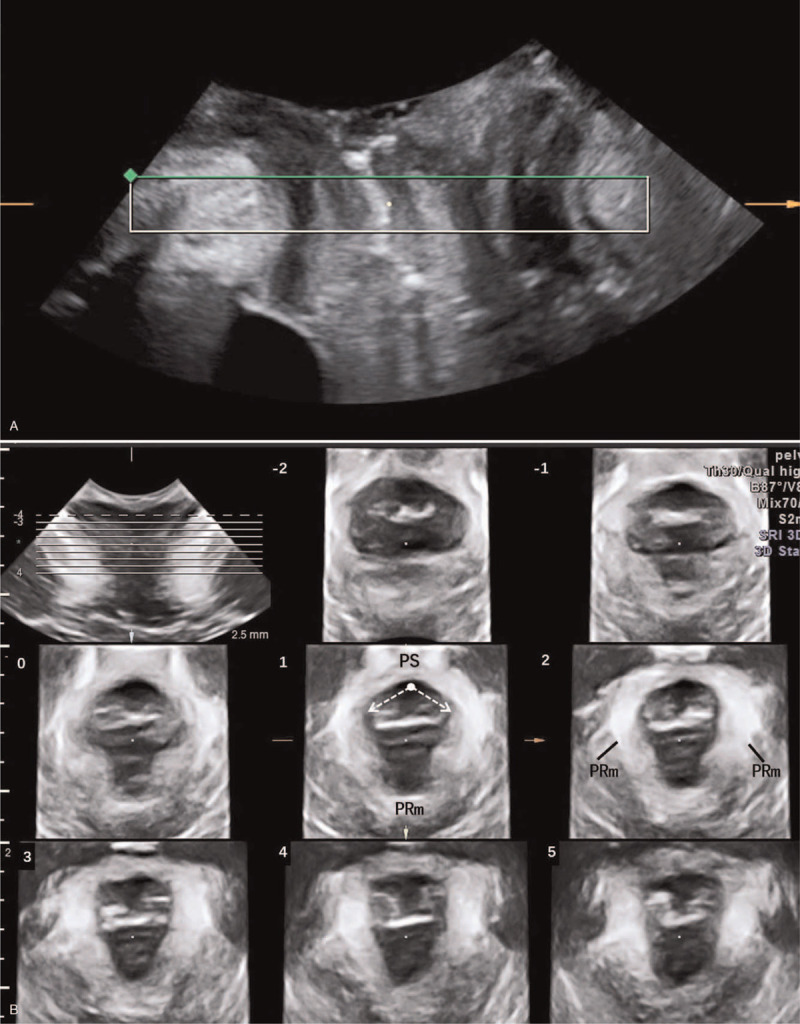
(A) In midsagittal plane, the plane of the minimal hiatal dimension is identified between the hypoechoic posterior margin of pubic symphysis and hyperechoic anterior border of puborectalis. (B) The perineal view of the levator hiatus obtained by tomographic ultrasound imaging (TUI), from slice -2 to slice 5. White arrows show the measurement of levator-urethra gap (LUG). PS, pubic symphysis; PRm, puborectalis.
